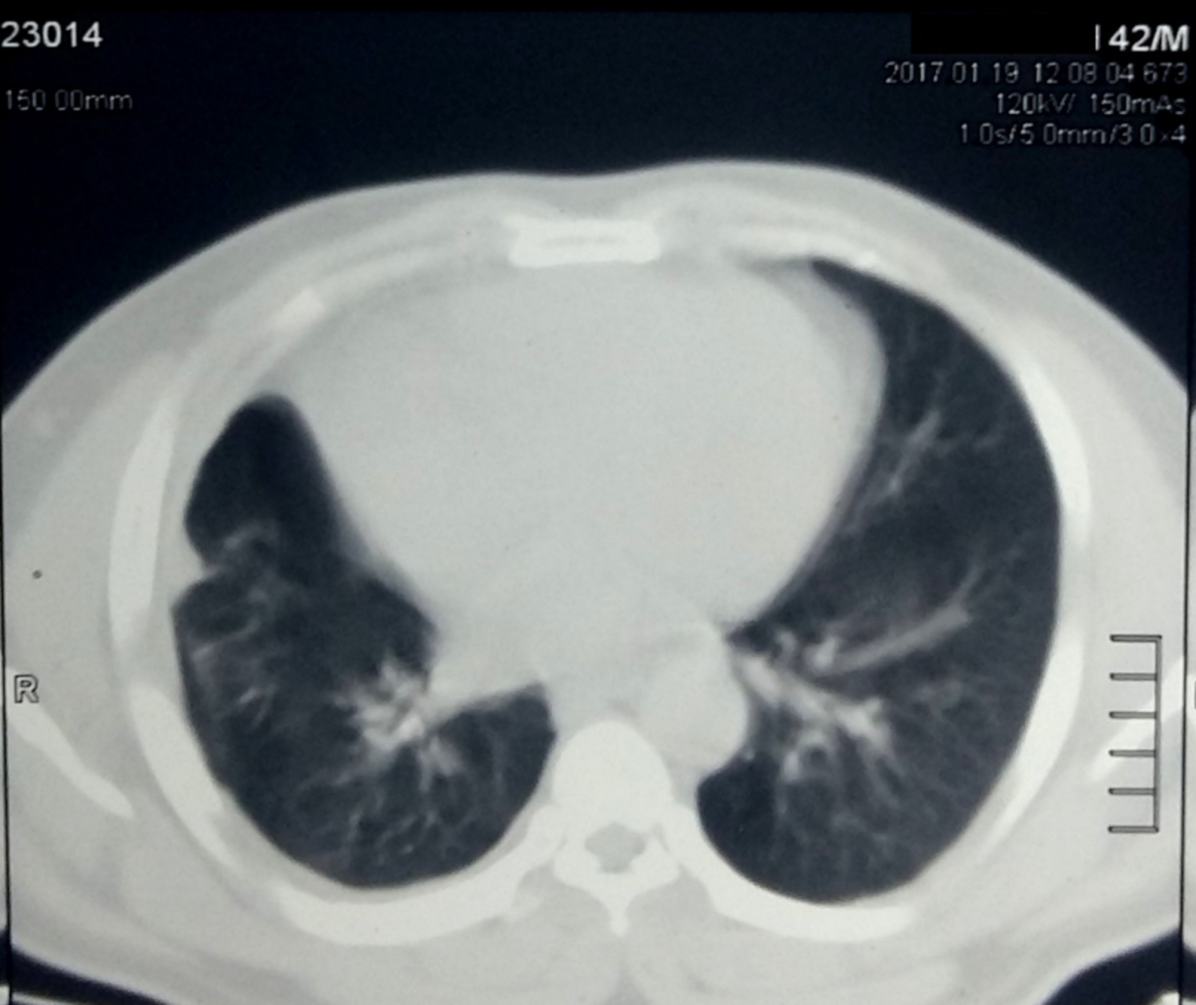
Computed tomography (CT) of the chest showing an absence of pleural or lung metastases
